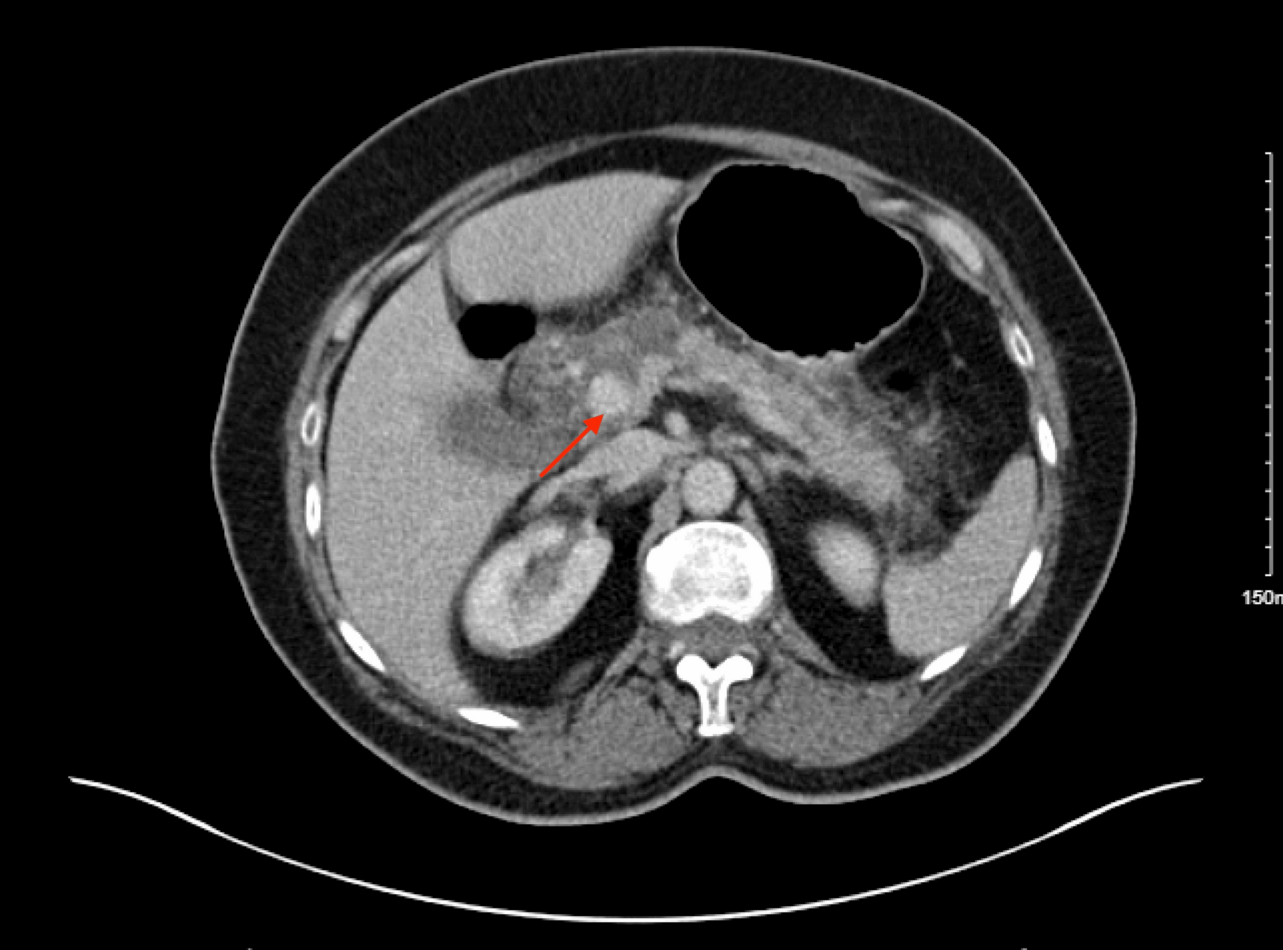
CT abdomen and pelvis showing fat stranding consistent with acute pancreatitis (red arrow). CT, computed tomography
